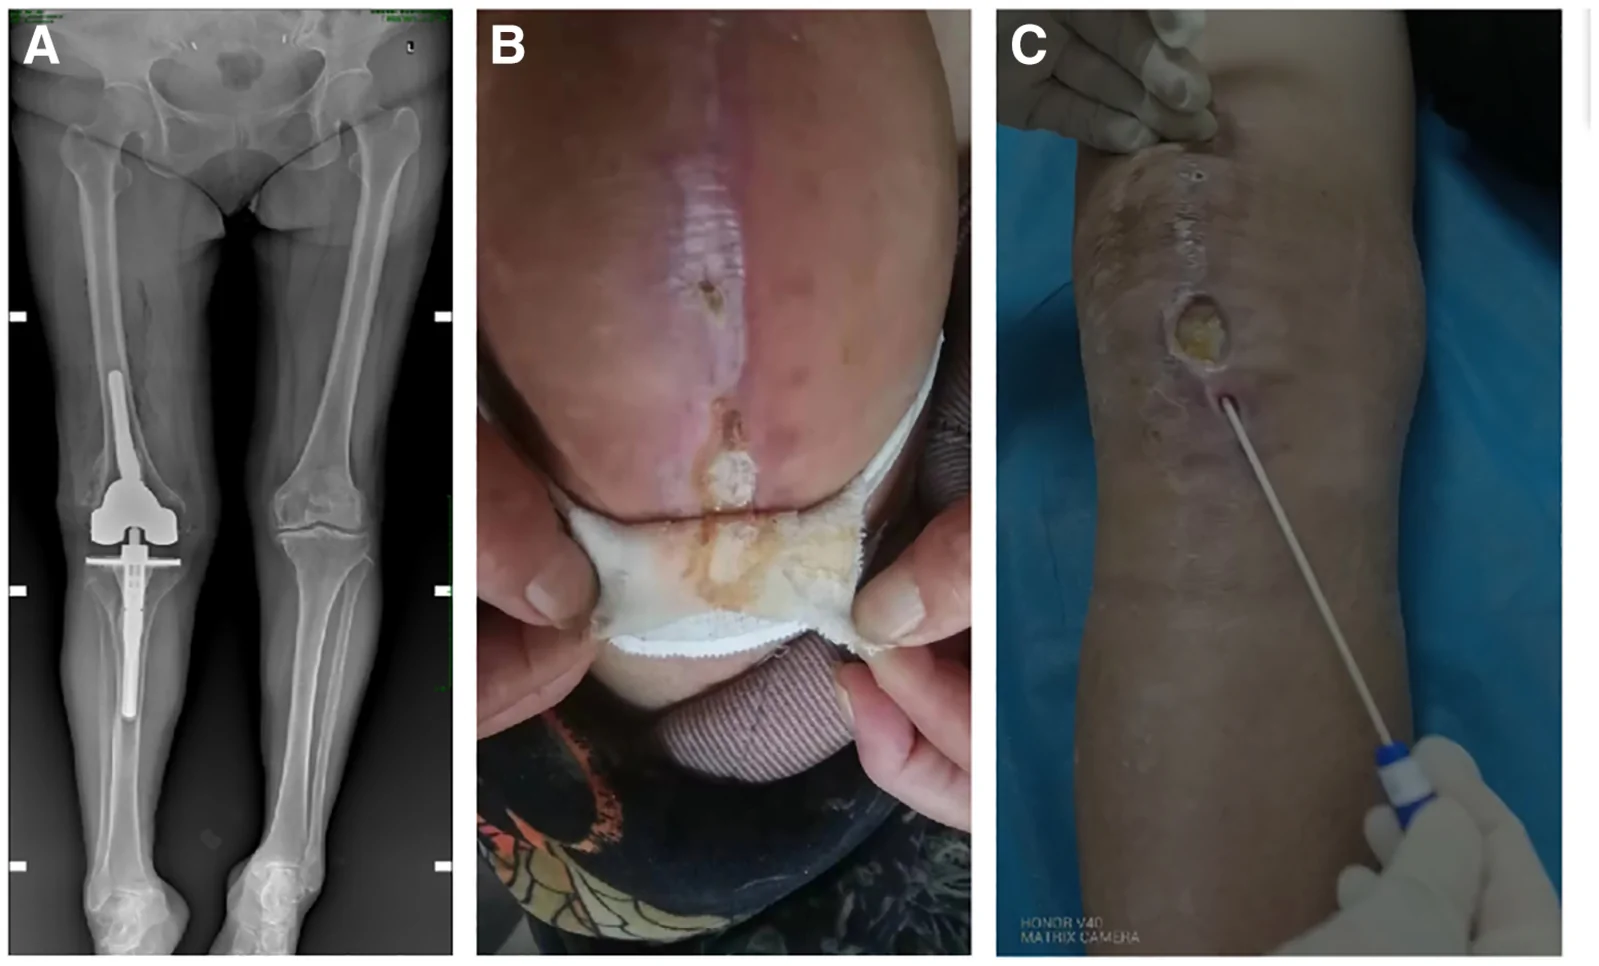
(A) Postoperative radiograph of full-length lower limb. (B) and (C) postoperative photos show poor wound healing at the incision site, ultimately leading to ulceration.
